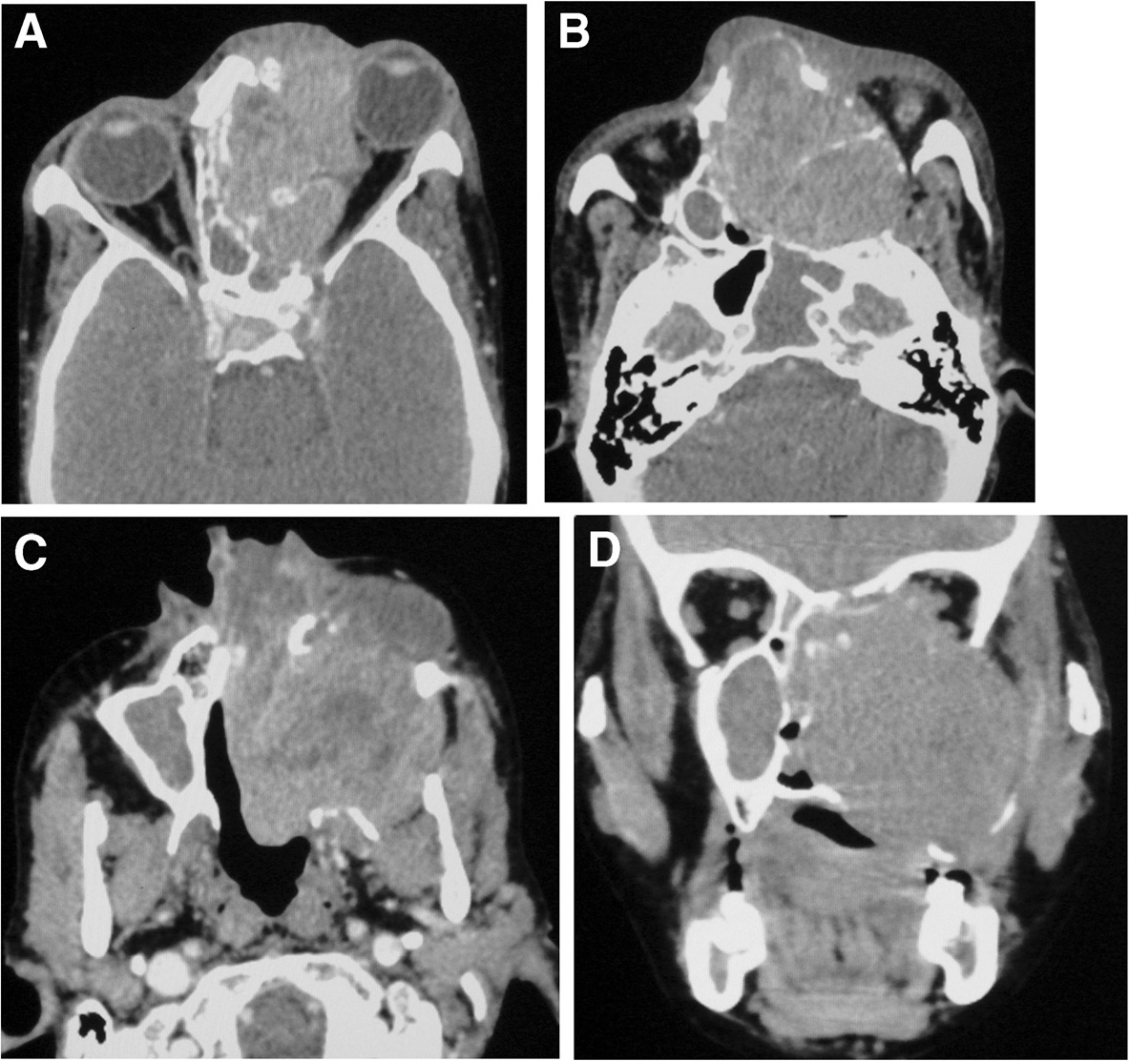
CT images in soft tissue window showing mass in ethmoid sinus and nasal cavity extending into the left orbit. A &B: Axial section CT image showing heterogeneously enhancing soft tissue density mass in bilateral ethmoid sinuses and both nasal cavities with bony destruction and extension into left orbit causing proptosis. C &D: Axial and coronal section CT images showing lateral extension of the mass into left infratemporal region with destruction of the postero-lateral wall of left maxillary sinus.
